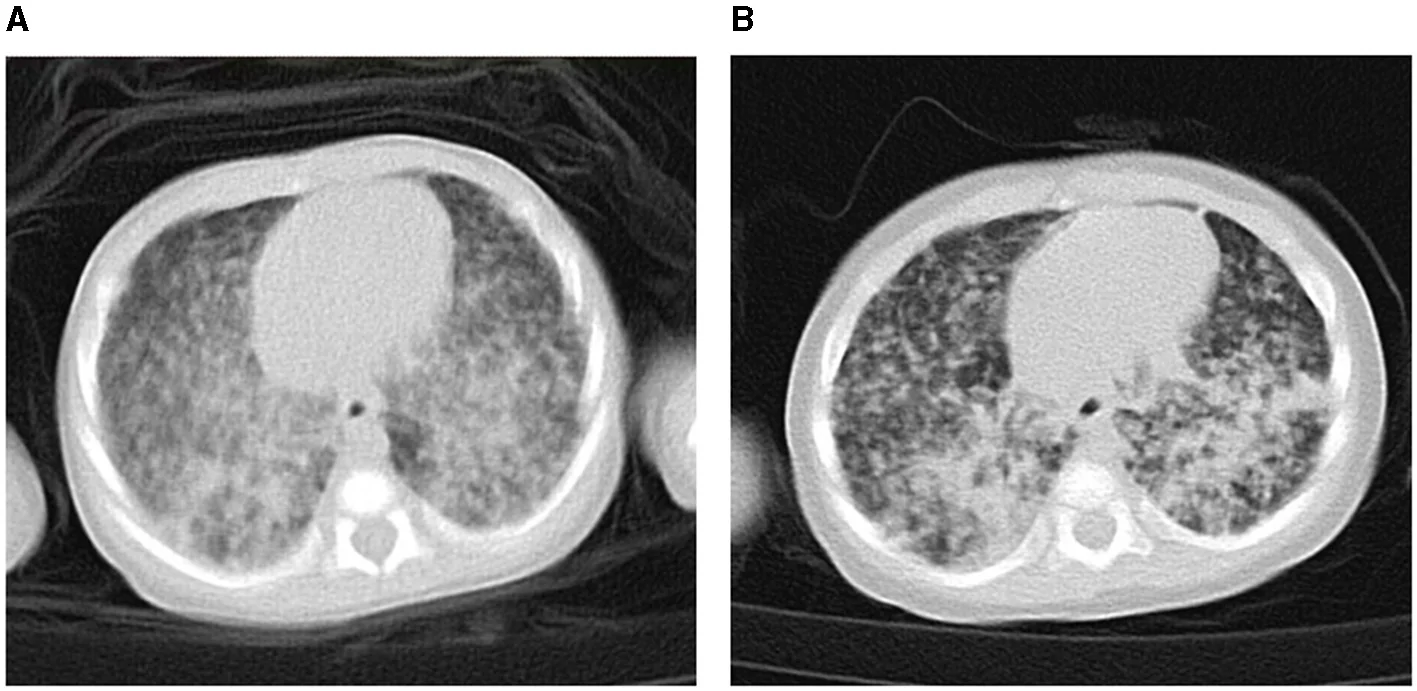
Chest computed tomography of the patient at diagnosis of congenital tuberculosis (TB). (A) On the 20th day of anti-TB treatment/day of life 41. (B) On the 70th day of anti-TB treatment/day of life 91.
